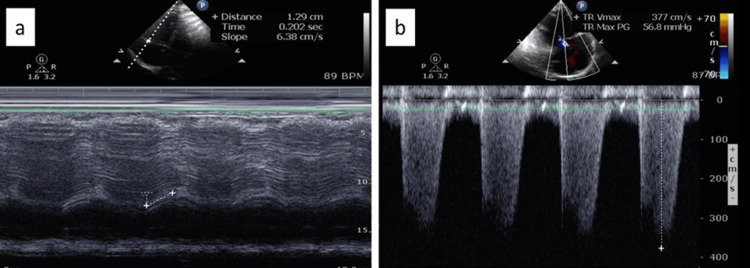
Transthoracic echocardiography (TTE) images showing the following: (a) right ventricular function measurement by tricuspid annular plane systolic excursion (TAPSE) low at 1.29cm (normal >1.7cm) and (b) tricuspid regurgitation velocity of 3.77cm/s. Calculated pulmonary artery systolic pressure (PASP) is severely elevated at 71.85mmHg PASP: Peak velocity square X 4 plus RA pressure; in this case: 3.77 squared X 4 plus 15 mmHg=71.85mmHg
